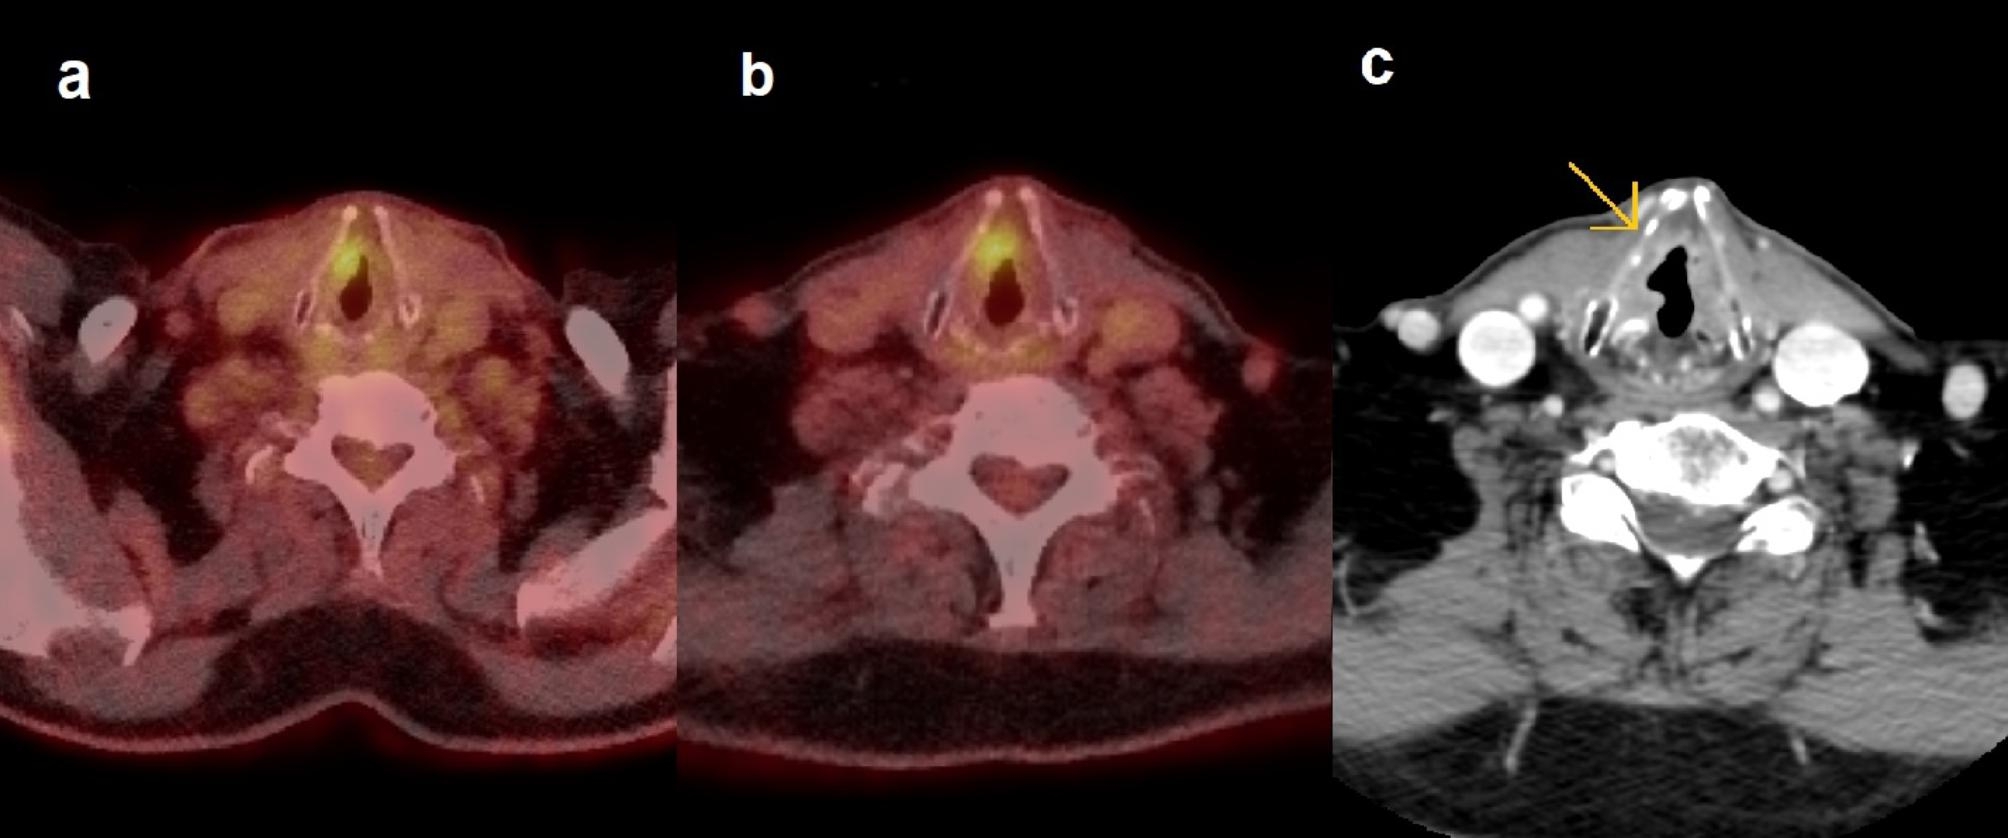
False positive result on two consecutive PET-CTs. On the PET-CT 3 months after the end of treatment, the area of the previous primary tumor around the right laryngeal ventricle showed a focal mild radiotracer uptake of SUV_max_ 3.2 (a). The laryngoscopy revealed a small bump on the anterior vocal cord, which revealed only mild chronic inflammation on biopsy. The PET-CT was repeated 9 months later because of hoarseness and an infectious-looking lesion on the false cord. Focal uptake on the previously detected uptake area had increased to 4.1 (b). On laryngoscopy, the false cord appeared exophytic, and biopsies showed epithelial hyperkeratosis and hyperplasia. Two and half years later (three and half years after end of treatment), the patient returned with a right-sided glottic tumor growth with biopsies resulting in SCC. CT (c) showed a malignant-looking enhancing lesion with ulceration on the anterior commissure, and anterior and middle thirds of the vocal cord
